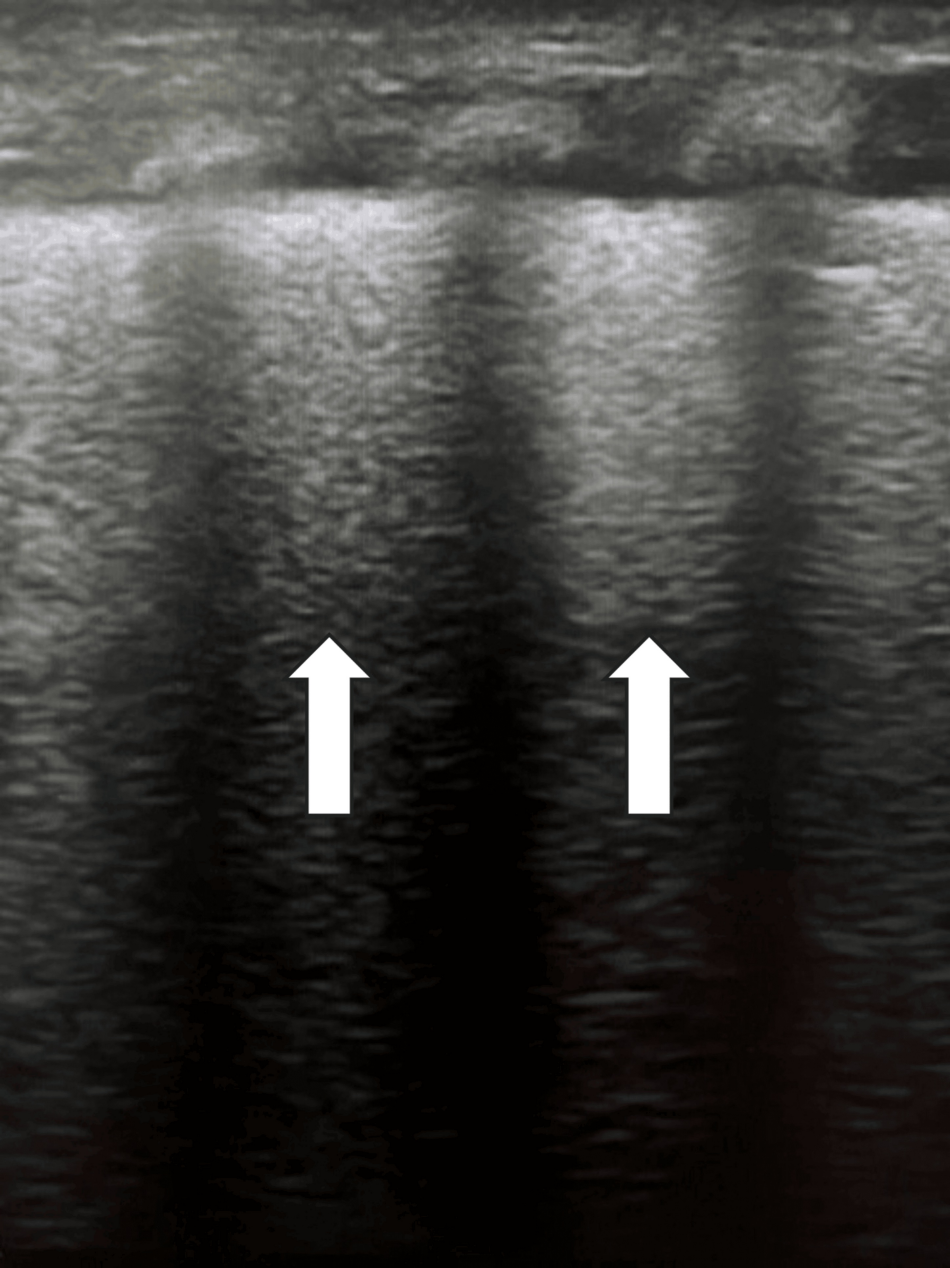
Neonate with hyaline membrane disease The lung ultrasound demonstrates a compact B-line pattern and the absence of an A-line pattern in the anterior superior lung field of a premature newborn of 29 gestational weeks, 30 minutes after the delivery.
